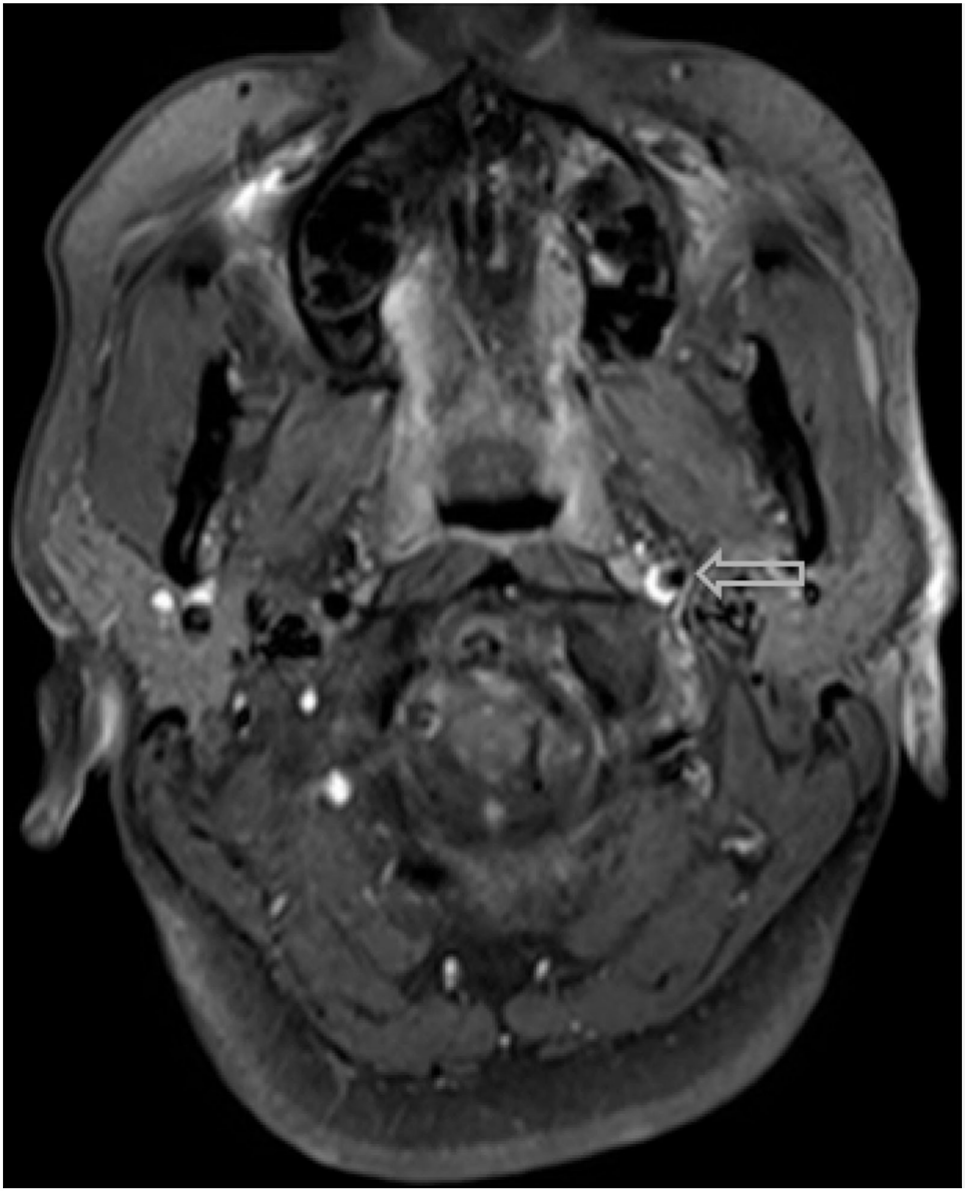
A hyper-intense, crescent-shaped signal noting the presence of an acute thrombus within the cranial arteries on MRI angiography.
